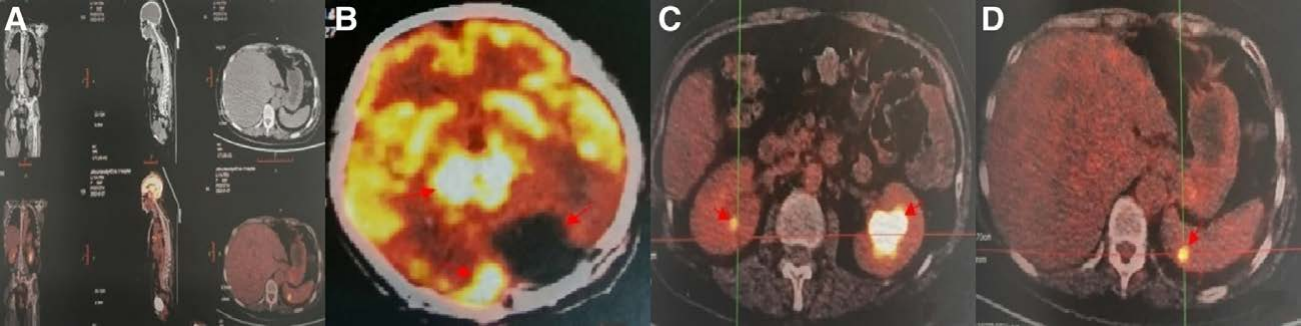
PET/CT showed normal distribution of skeletal radioactivity throughout the body (A), no abnormal radioactive uptake in the left cerebellar curtain region and hypermetabolic foci in the falx and pineal regions (B). angiosmooth muscle lipoma in the right kidney, hydronephrosis in the left kidney (C) and hypermetabolic nodules in the spleen (D). PET/CT = positron emission tomography/computed tomography.
